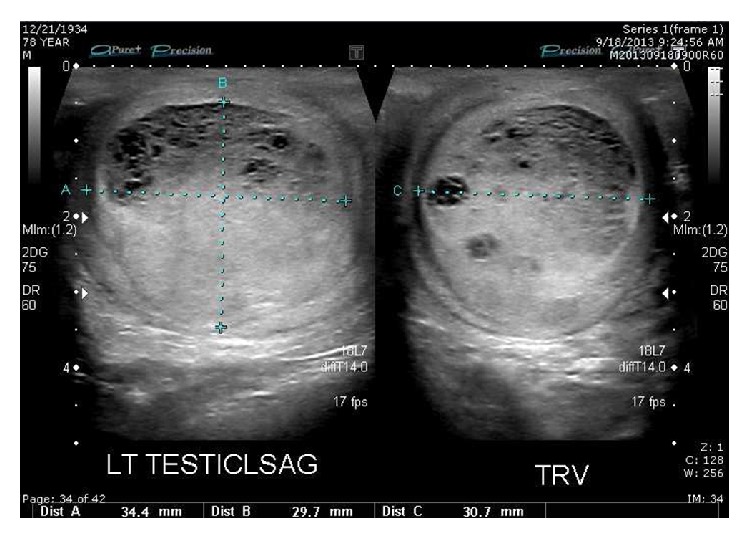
Scrotal ultrasound of left testis (sagittal and transverse views). Note the cystic and solid lesion of mixed echogenicity.
